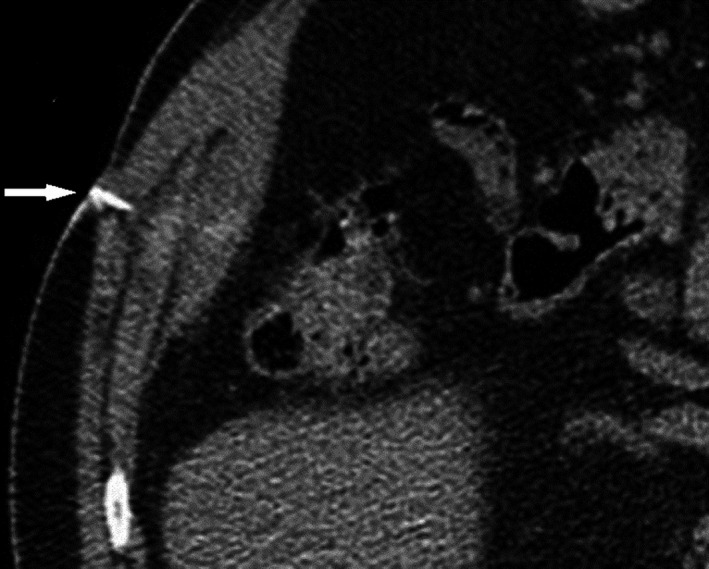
Axial section of contrast enhanced CT, with sinogram probe (arrow) within the tract, and no evidence of filling defect or side‐branching of tract.
